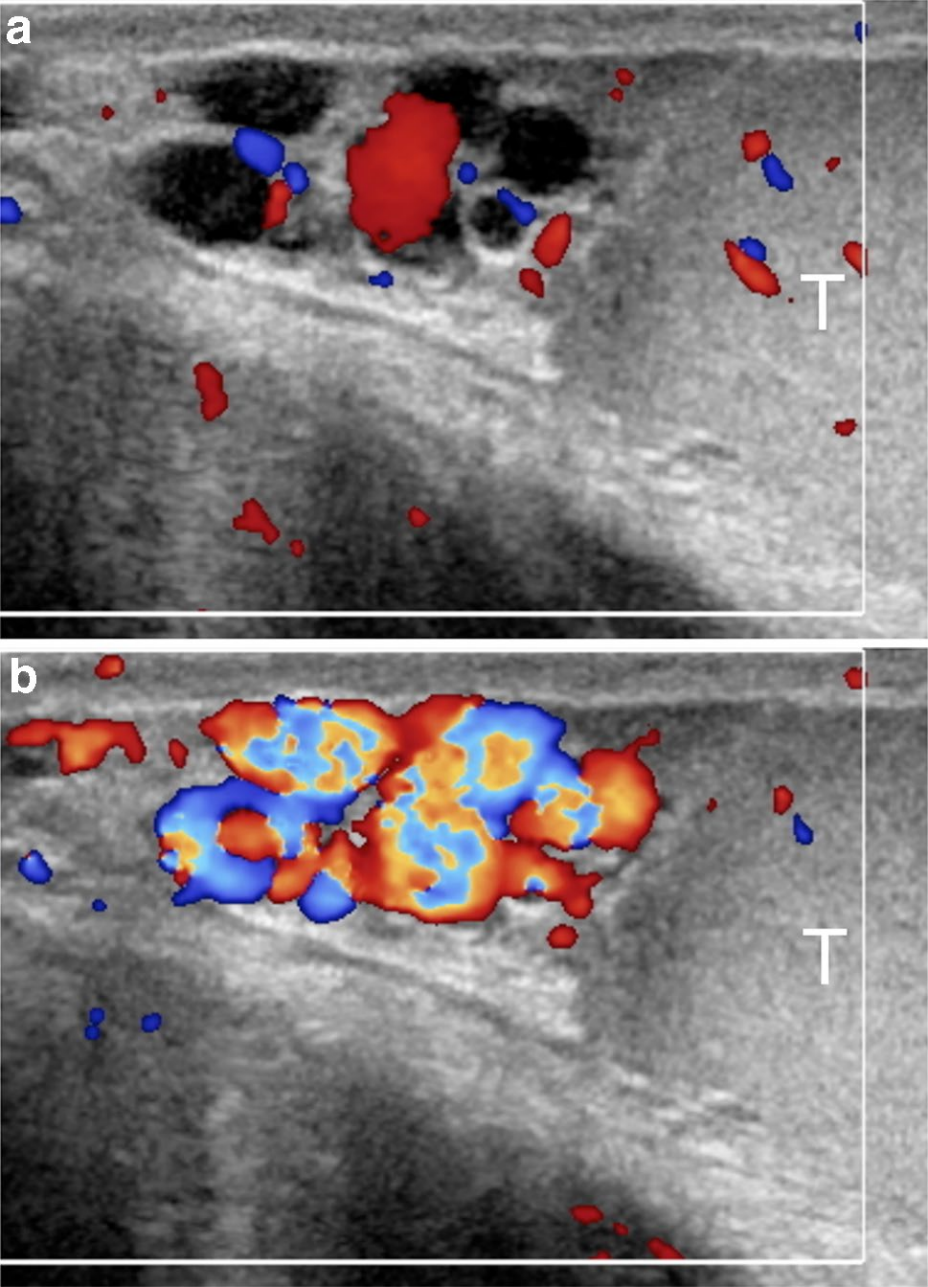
Sarteschi’s grade II varicocele. Colour Doppler images obtained at rest (a) and during Valsalva (b) showing dilated veins in the supratesticular region with reflux during Valsalva (T = testis)
